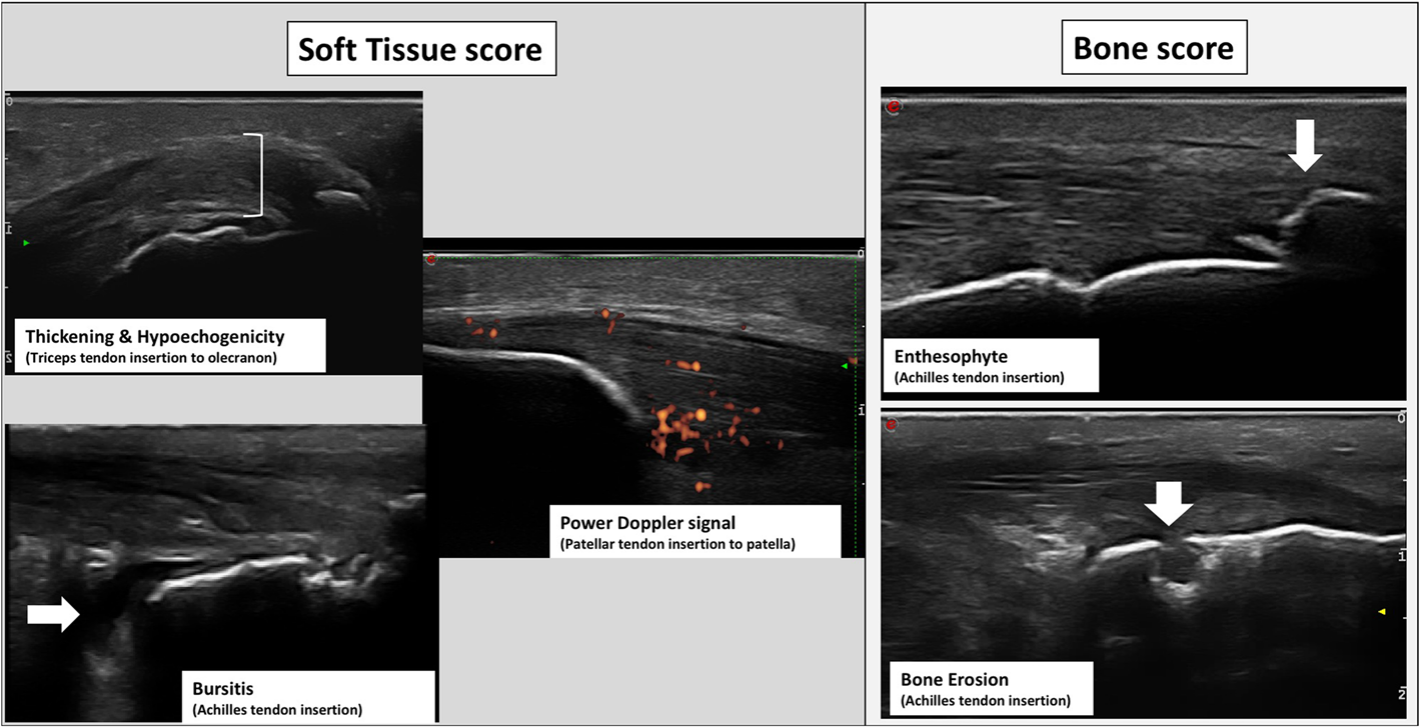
Elementary lesions of enthesitis included in the Madrid Sonography Enthesitis Index (MASEI)
